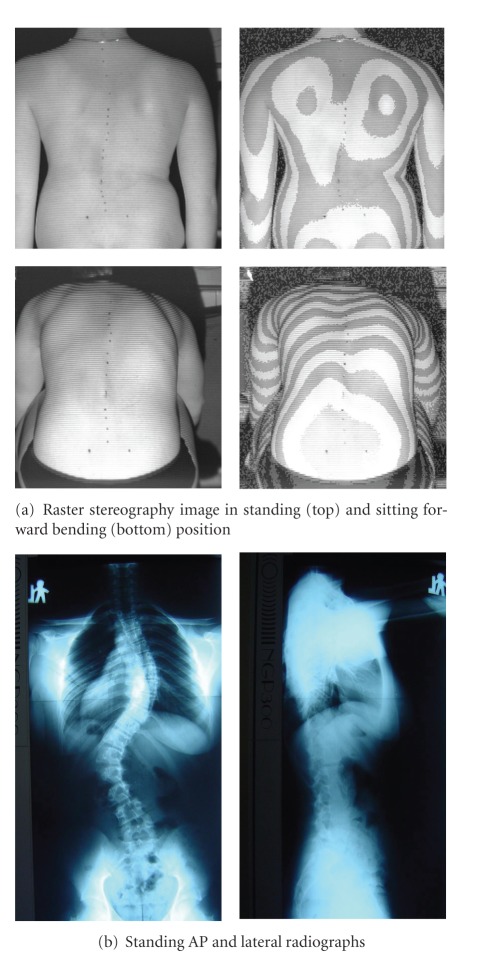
16 year old girl, treated with Cheneau brace for 1.5 years, including 5 months full time and 1.0 year part time wearing. Thoracic Cobb angle 54°, lumbar Cobb angle 60°, Risser sign 4. Two years after menarche. Main curve ATR = 10°, sum of three ATRs = 17°, POTSI = 16.0, HS = 14, (a) raster stereography; (b) radiographs.
